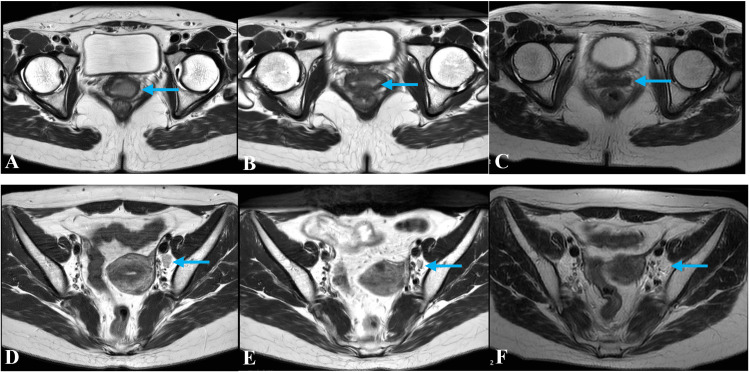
Pelvic MRI images of the patient. A, D: Pelvic MRI T2 images of the patient before treatment. B, E: Pelvic MRI T2 images of the patient after EBRT. C, F: Pelvic MRI images of the patient one month after treatment. MRI: magnetic resonance imaging; EBRT: external-beam radiotherapy
